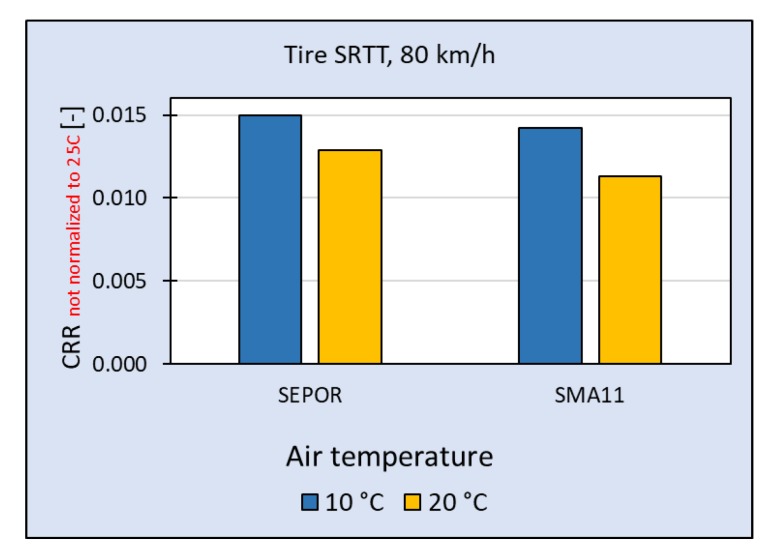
Coefficient of Rolling Resistance for SEPOR-G-PSMA5 W4 and reference pavement SMA11 measured at the Galaktyczna section for tire Avon AV4; values not normalized to 25 °C.
